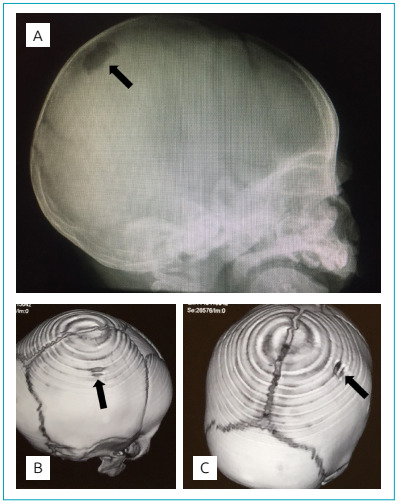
X-ray (A) and 3D computed tomography images (B and C) of the skull of the infant with myiasis. The images show lytic lesions in the parietal bone, confirming the osteomyelitis of the external surface of the skull bone.
